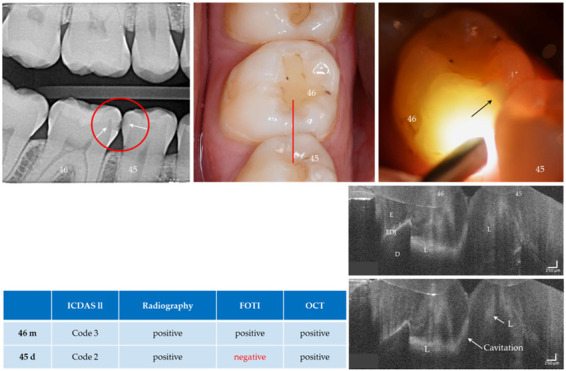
Teeth 46 (m) and 45 (d). Both surfaces showed visual and radiographic caries lesions (L, arrows) and dentin involvement was visible on tooth 46. The FOTI showed no signal on tooth 45 distally (arrow indicates the lesion of tooth 46), while a weak signal was visible on OCT. In the OCT images of tooth 46, dentin involvement is clearly visible and cavitation can be assumed due to the diagonal signal line (see Fig. 5b) and the anatomy of the tooth surface. *Verification*: Both the early carious lesion on tooth 45 and the cavitated lesion on tooth 46 could be confirmed later during the restoration of tooth 46. *Enamel (E), dentin (D), enamel-dentin junction (EDJ). The vertical scales are related to refractive index n = 1.5*.
